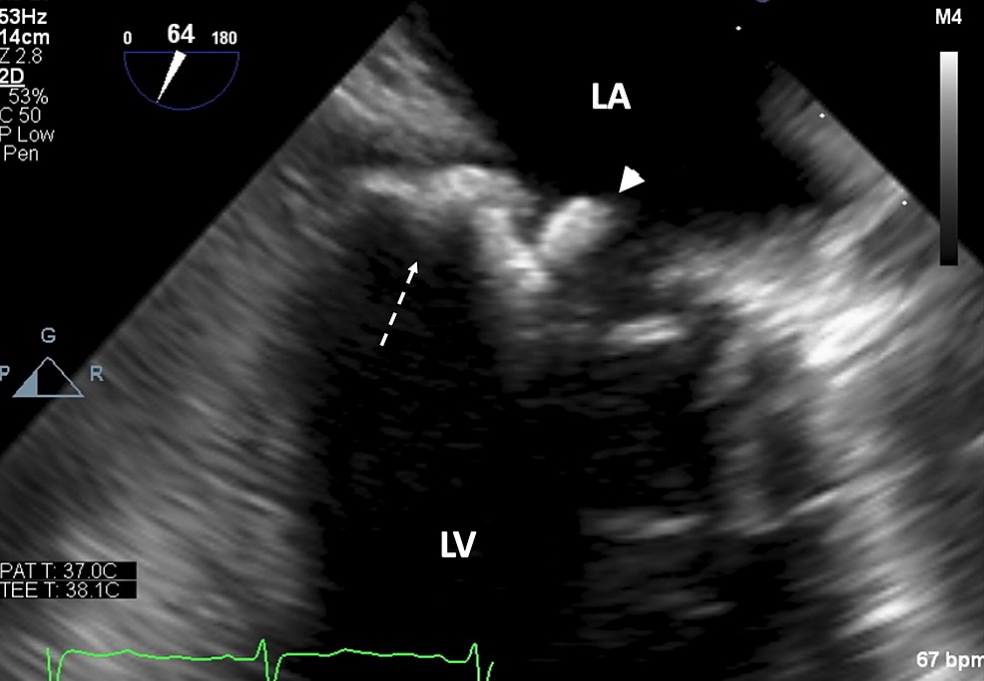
Transesophageal mid-esophageal view at 64 degree orientation showing both the MAC (broken arrow) and the caseous mass (arrowhead). LA = left atrium, LV= left ventricle, MAC = mitral annular calcification.
